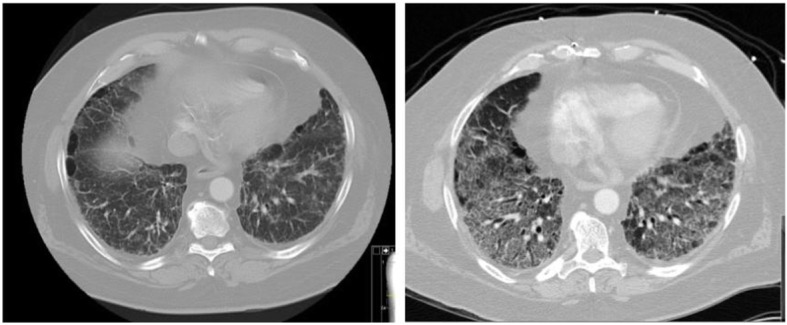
HRCT of CPFE patient. Baseline (left) and during AE-IPF (right). Findings include GGOs and interlobular septal thickening
